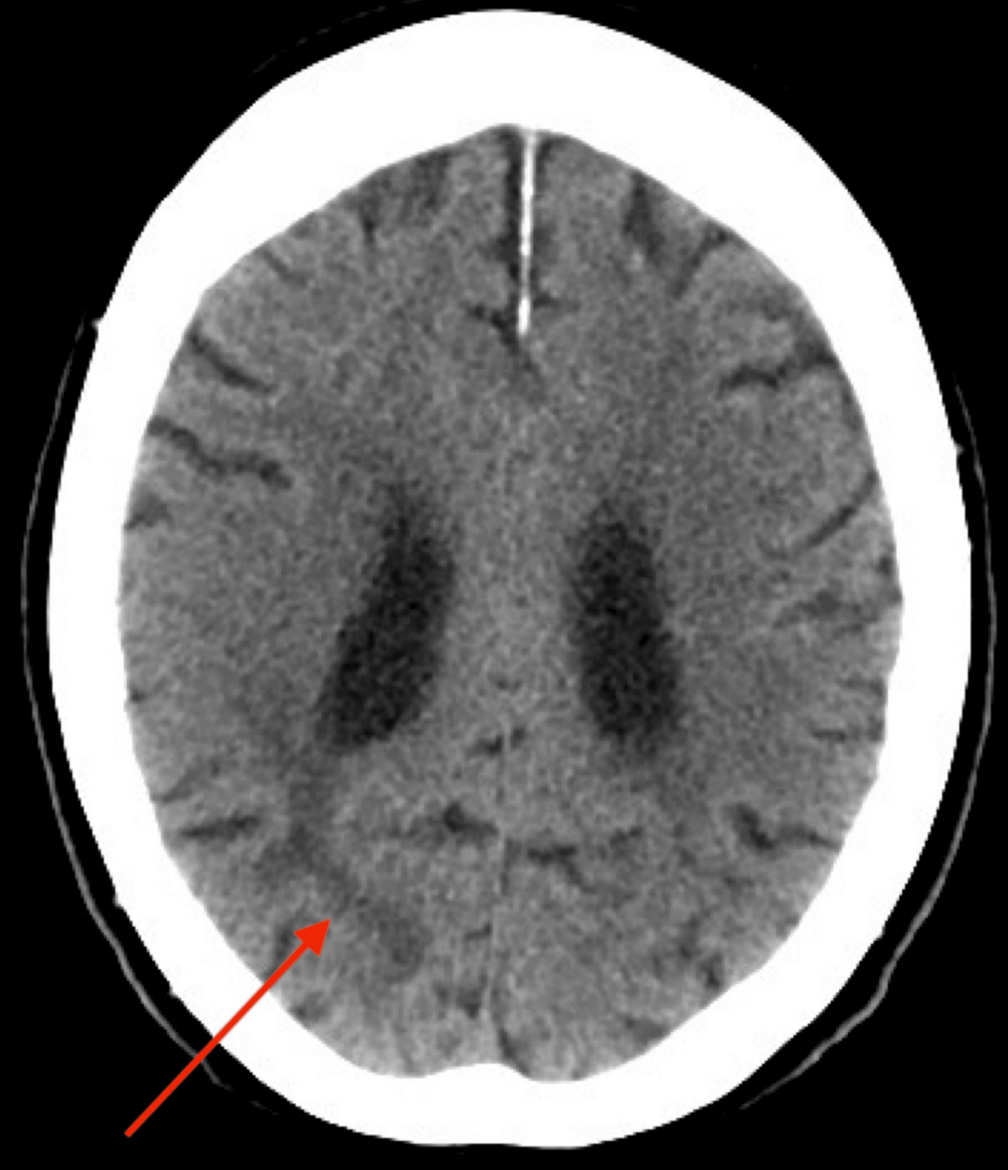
Right occipital lobe encephalomalacia Non-contrast axial CT scan of the brain showing an area of encephalomalacia in the right occipital lobe (red arrow), suggestive of a remote infarct.
